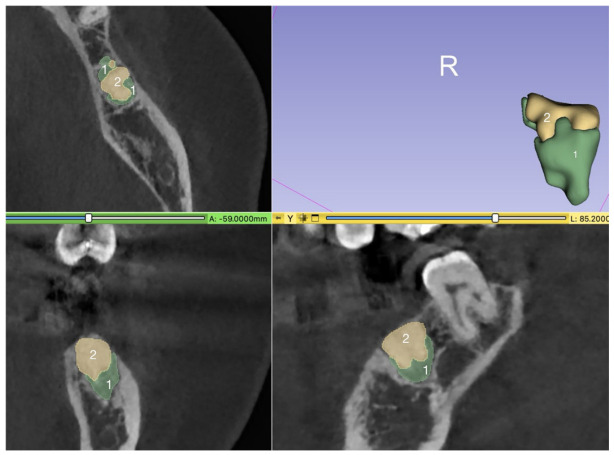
(1) “Socket” and (2) “Bio-Oss Collagen (xenograft)” within the three dimensions of the CBCT. The 3D model is presented in the upper right corner.
